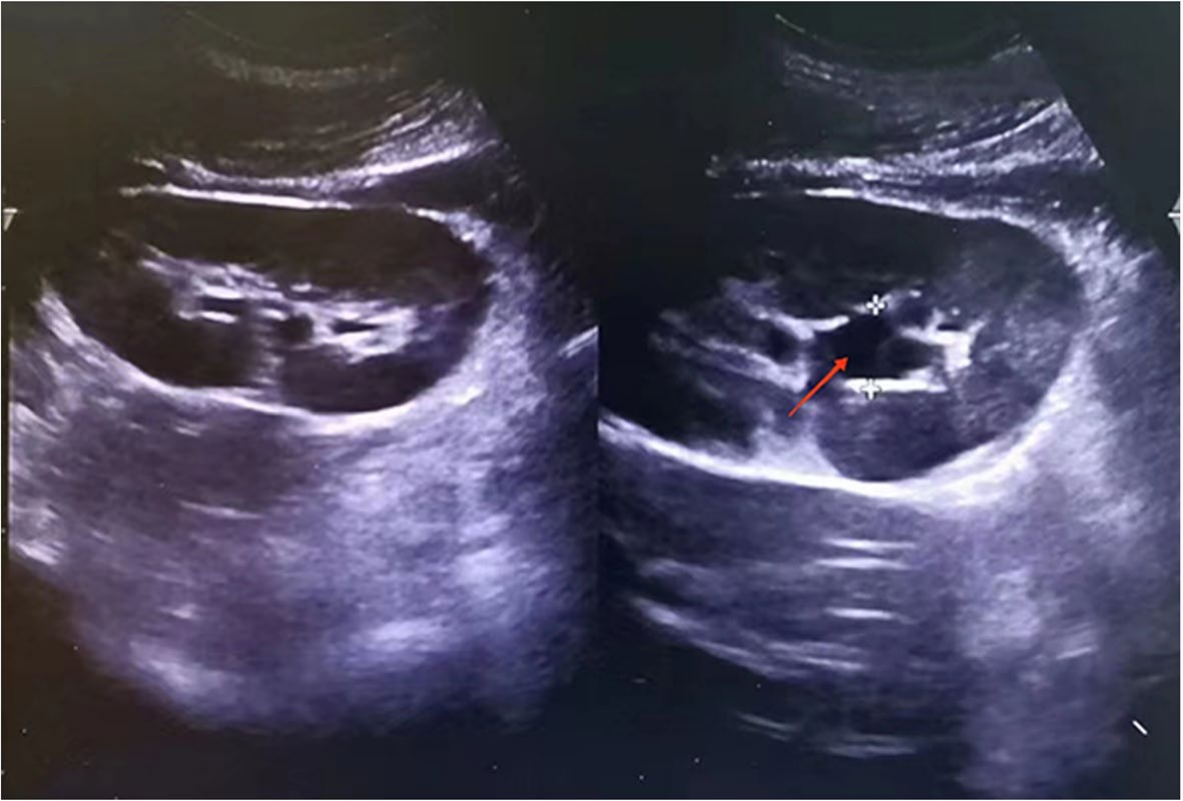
Ultrasound of both kidneys showed separation of the renal pelvis and calyces with a width of approximately 17 mm at the widest point (red arrow)
